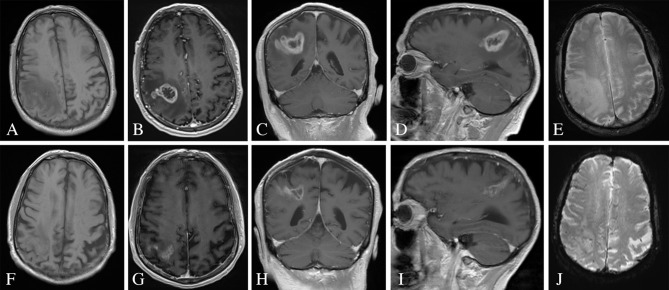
MRI of the brain pre- and post-corticosteroid therapy. (A) Initial T1-weighted imaging without evidence of subacute hemorrhage. (B–D) Initial MRI MP-RAGE sequences of the brain pre-corticosteroid therapy demonstrated a thick-walled peripherally enhancing lesion of the right parietal lobe with central necrosis and extensive surrounding vasogenic edema. (E) Initial gradient echo sequence without evidence of subacute hemorrhage. (F) Repeat T1-weighted imaging without evidence of subacute hemorrhage. (G–I) Repeat MRI MP-RAGE sequences of the brain 5 weeks after post-corticosteroid therapy demonstrated reduction in contrast enhancement, vasogenic edema, and apparent size of the lesion. (J) Repeat gradient echo sequence without evidence of subacute hemorrhage.
